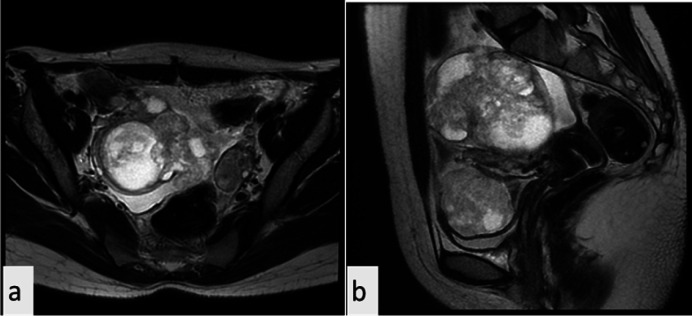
MRI study: a. Sagittal T2 images. b. AxialT2 images
